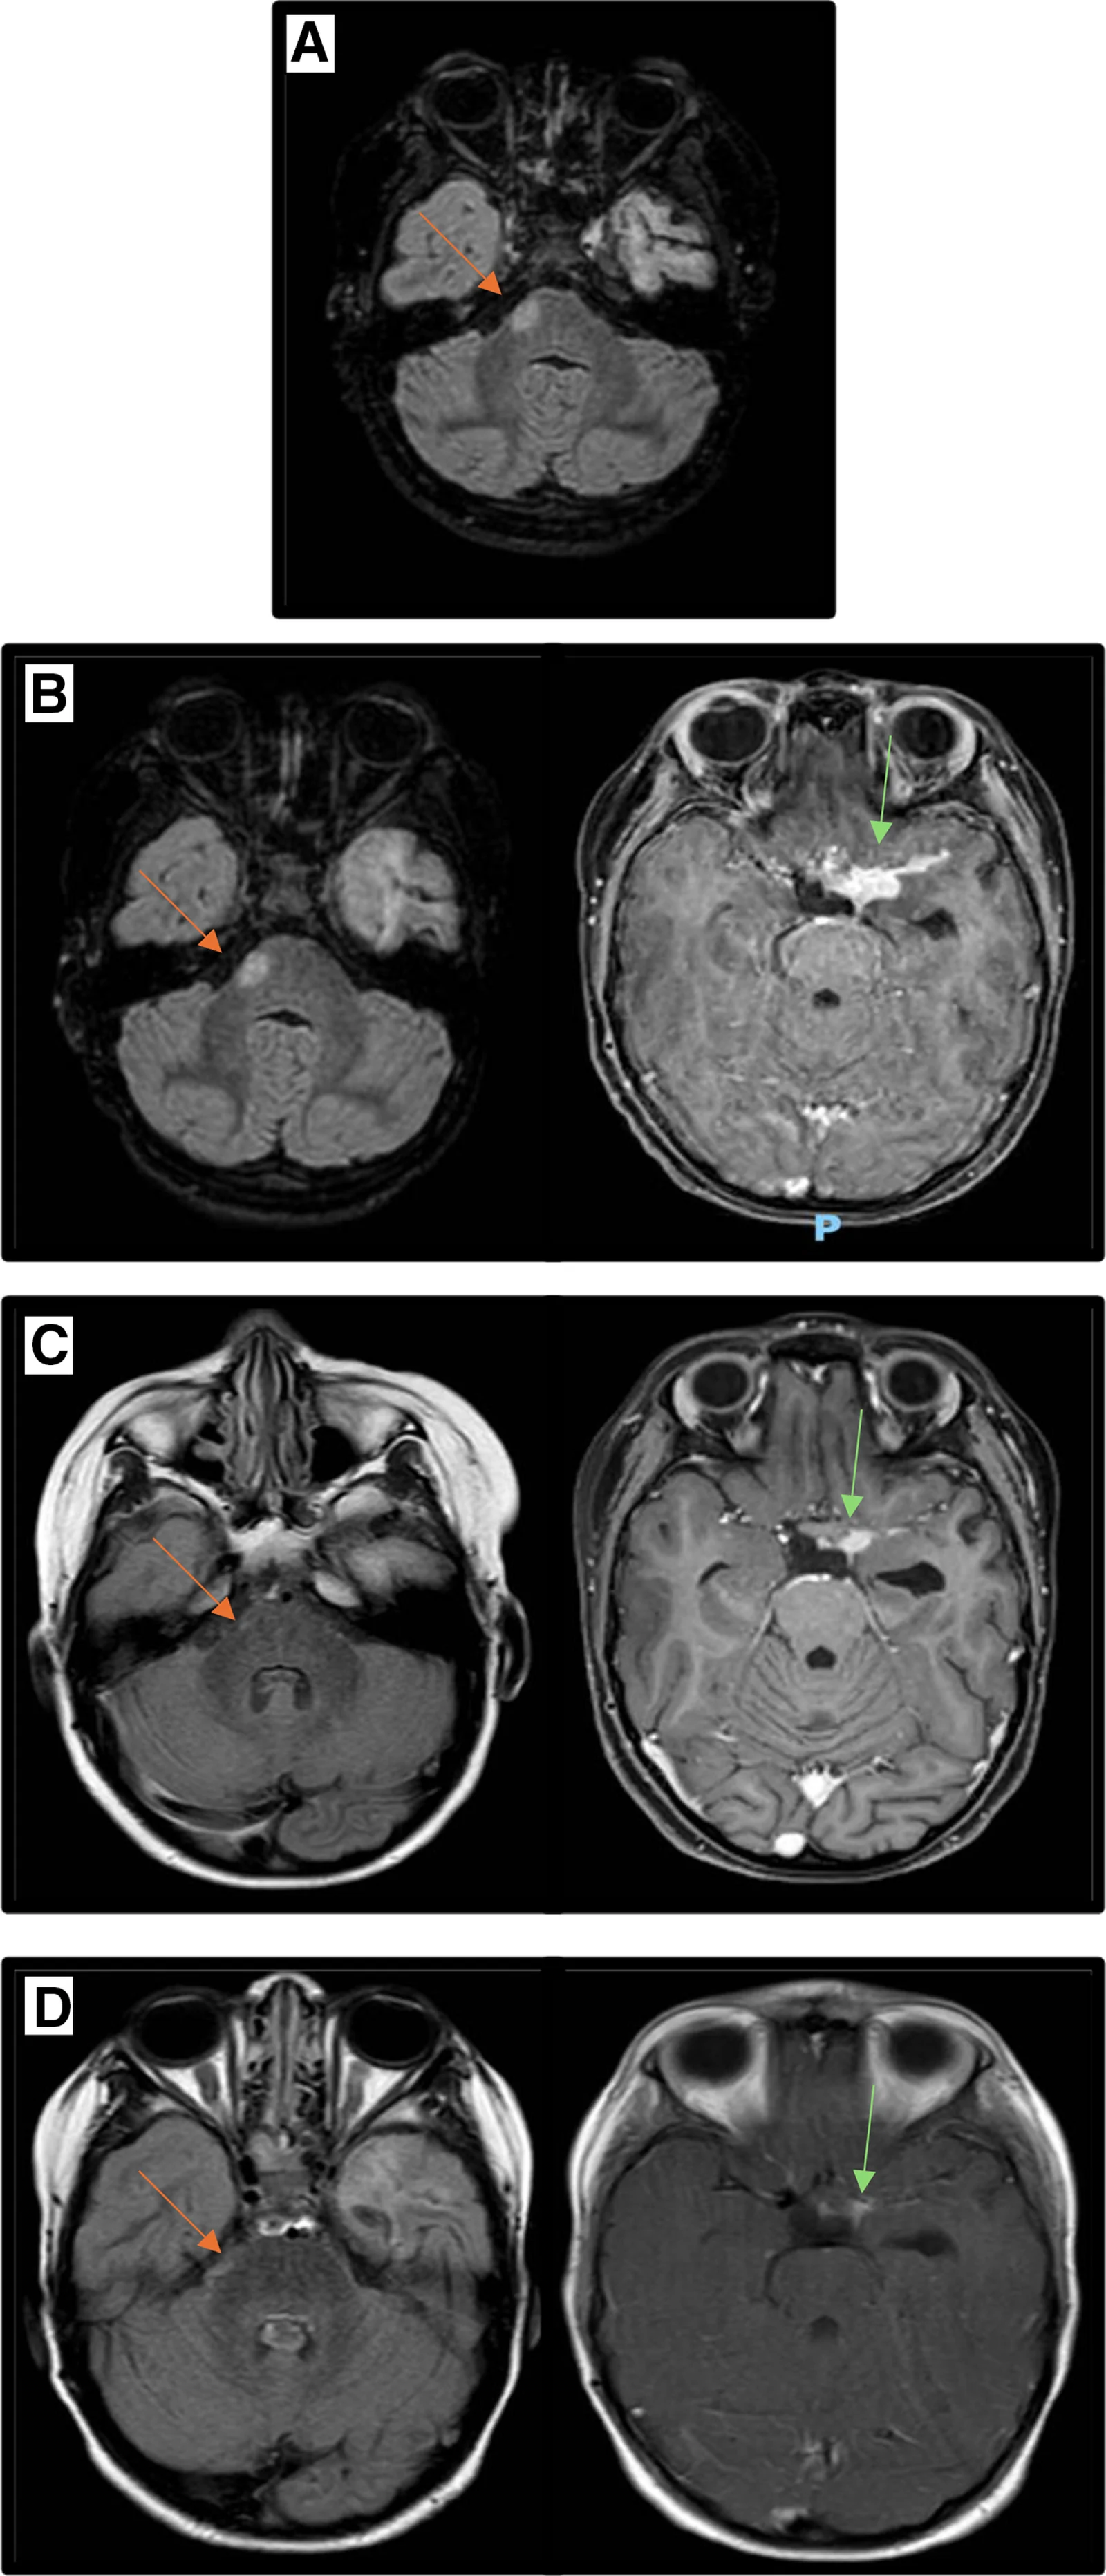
A: Transverse MRI brain sequences (axial 3D Flair) performed on day 60 (after 58 days of antitubercular therapy), showing gliotic-malacic evolution in both cerebral hemispheres, consistent with ischemic damage secondary to the initial vasculitic insult, along with the appearance of a contrast-enhancing nodule (<5 mm) protruding into the right prepontine cistern. B: Transverse MRI brain sequences (axial 3D Flair) performed at day 88 (after 72 days of nonconsecutive antitubercular therapy, discontinued for 14 days due to hepatotoxicity), showing stable gliotic-malacic changes in the caudate, putamen, thalamus, and nucleo-capsular regions, increased extent of edema in the left temporopolar and frontobasal cortico-subcortical regions, a slight increase in size (~5 mm) of the previously described contrast-enhancing nodule in the right prepontine cistern and the new appearance of a 7 mm ring-enhancing nodule in the left temporopolar region, consistent with a caseating granuloma (sT1W 3D Wats). C: Transverse MRI brain sequences, performed on day 110 (after administration of first and second dose of infliximab), showing a marked reduction in edematous changes in the left temporopolar and frontobasal cortico-subcortical regions, replaced by gliotic-malacic changes with initial signs of tissue loss; a reduction in size of the previously described ring-enhancing lesion adjacent to the basal cisterns (sT1W 3D Wats sequence, 5 mm vs. 7 mm in the previous report); and an almost complete resolution of the hyperintense T2/FLAIR lesion in the right lower pons. The previously enlarging nodular contrast-enhancing (CE) lesion in the adjacent cistern is now almost resolved, appearing as a pinpoint lesion. D: Transverse MRI brain sequences, performed on day 202 (3 months after discharge), showing continued regression of the infectious/inflammatory tissue within the left cisternal compartment. The previously reported round-shaped, T2/FLAIR hyperintense lesion with ring enhancement, is no longer appreciable. The punctate enhancement in the right pontomesencephalic cistern is resolved. MRI indicates magnetic resonance imaging.
